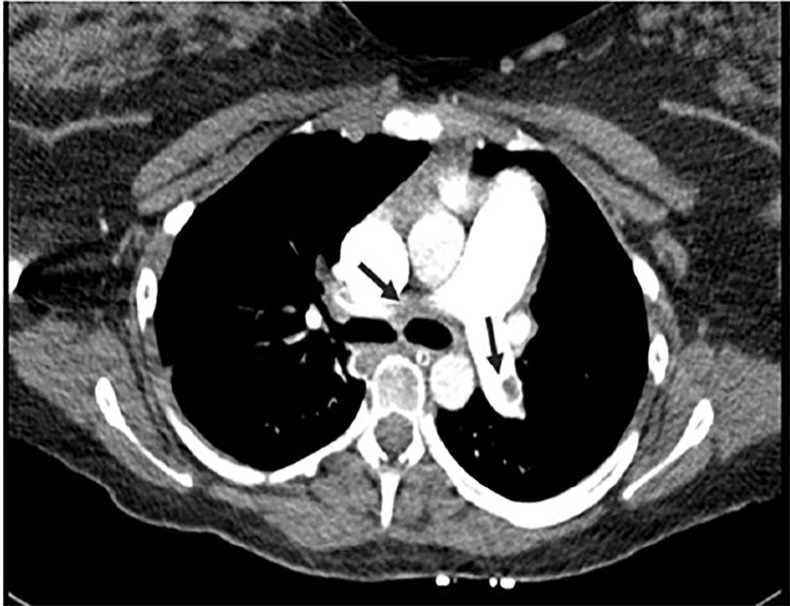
Computed tomography pulmonary angiogram showing bilateral large filling defects involving the main pulmonary arteries (arrows).
